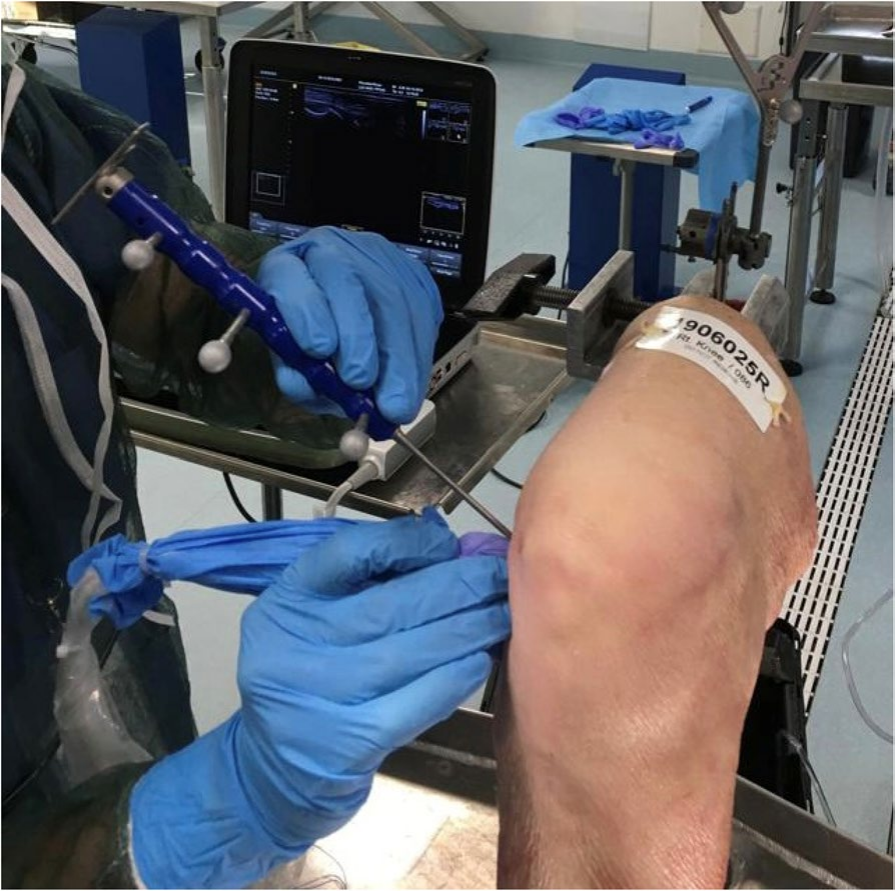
Anatomic ALL insertions assessed by ultrasonography
